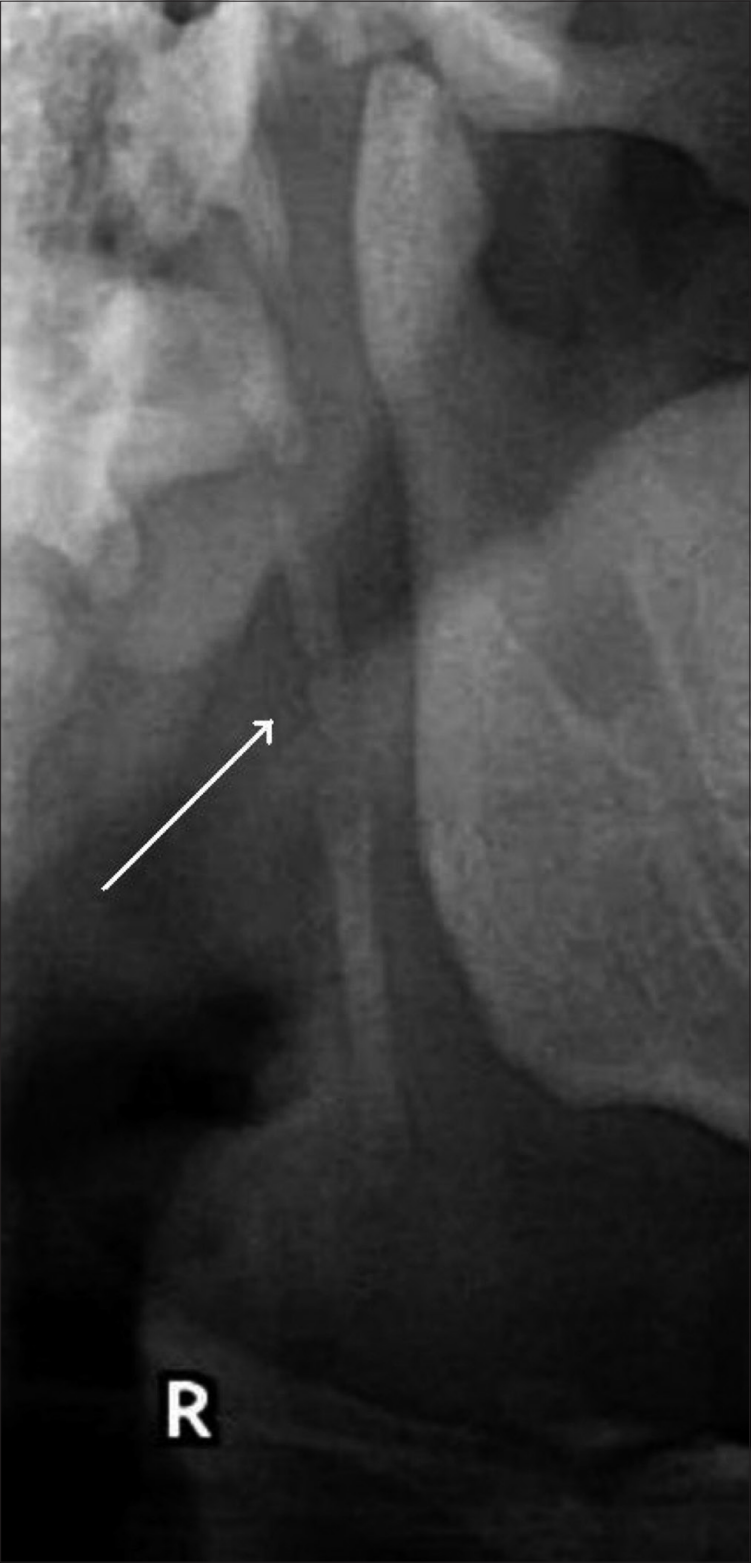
The orthopantomogram shows a Langlais type III styloid process (arrow)
